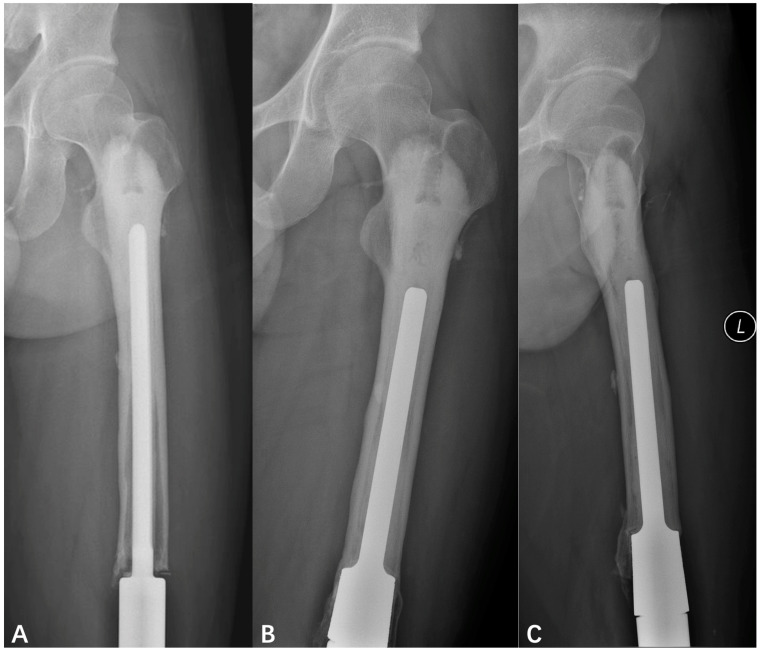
(A) AP radiographs of a 17-year-old man showed aseptic loosening at 75 months follow-up after prosthesis replacement. (B) The AP radiographs at 78-month follow-up post-revision showed no aseptic loosening and extracortical bone ingrowth in both the medial and the lateral aspects. (C) The lateral radiographs at 78-month follow-up post-revision showed no aseptic loosening and extracortical bone ingrowth in the posterior aspects but not the anterior aspect.
